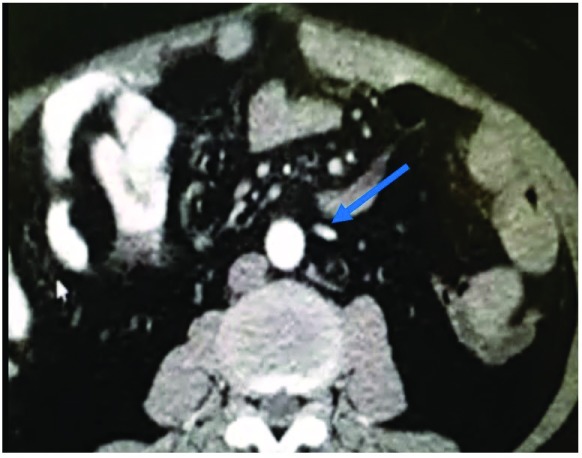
Abdominopelvic CT scan with IV contrast. Narrow enhancement of the inferior mesenteric artery can be observed (blue arrow).
